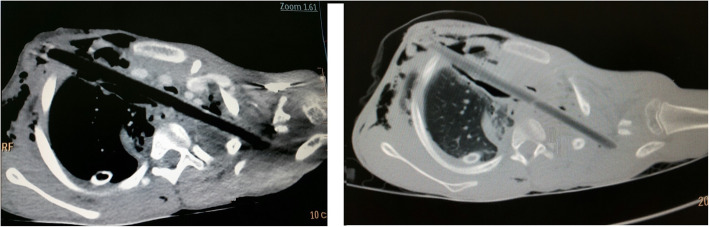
CT scan demonstrating the trajectory of the penetrating foreign body in the axial plane through the anterio-superior mediastinum, directed superiorly to the left side all the way to the medial edge of contralateral shoulder joint
